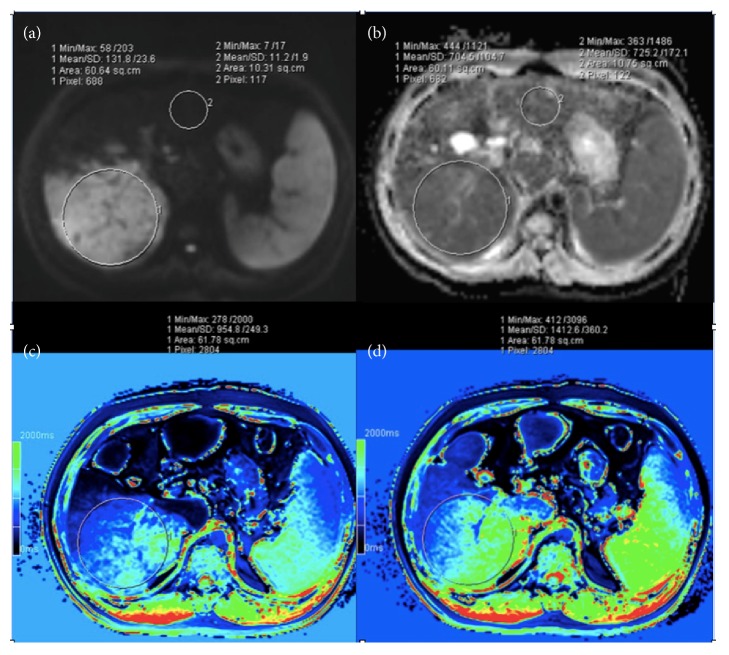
(a–d) Examples of placement of regions of interest (ROIs) in the same area of HCC lesion (ROI 1) and liver parenchyma (ROI 2) on a high-*b*-value DWI (a) and corresponding ADC maps (b) and ROIs of HCC lesion on T1 map before (c) and after (d) Gd-EOB-DTPA injection.
